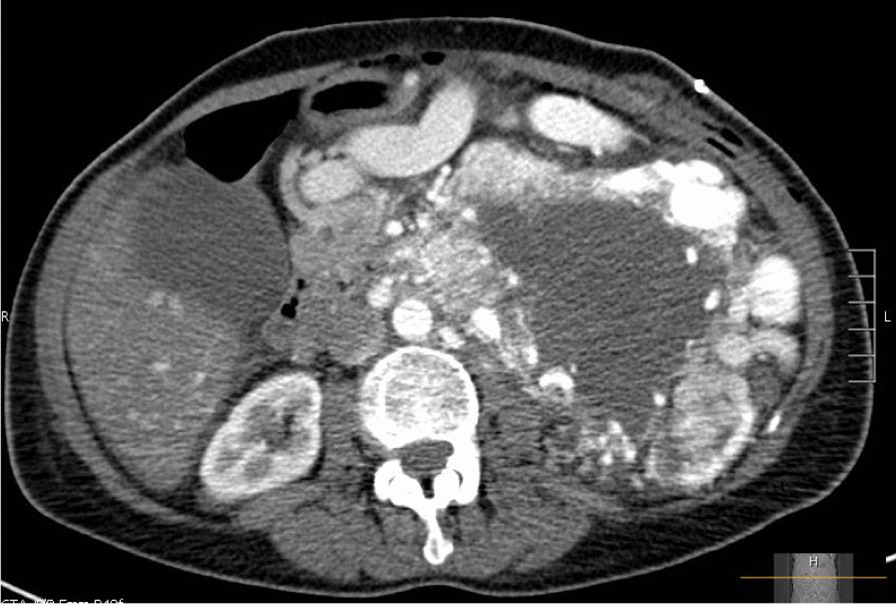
CT scan demonstrating left renal mass
